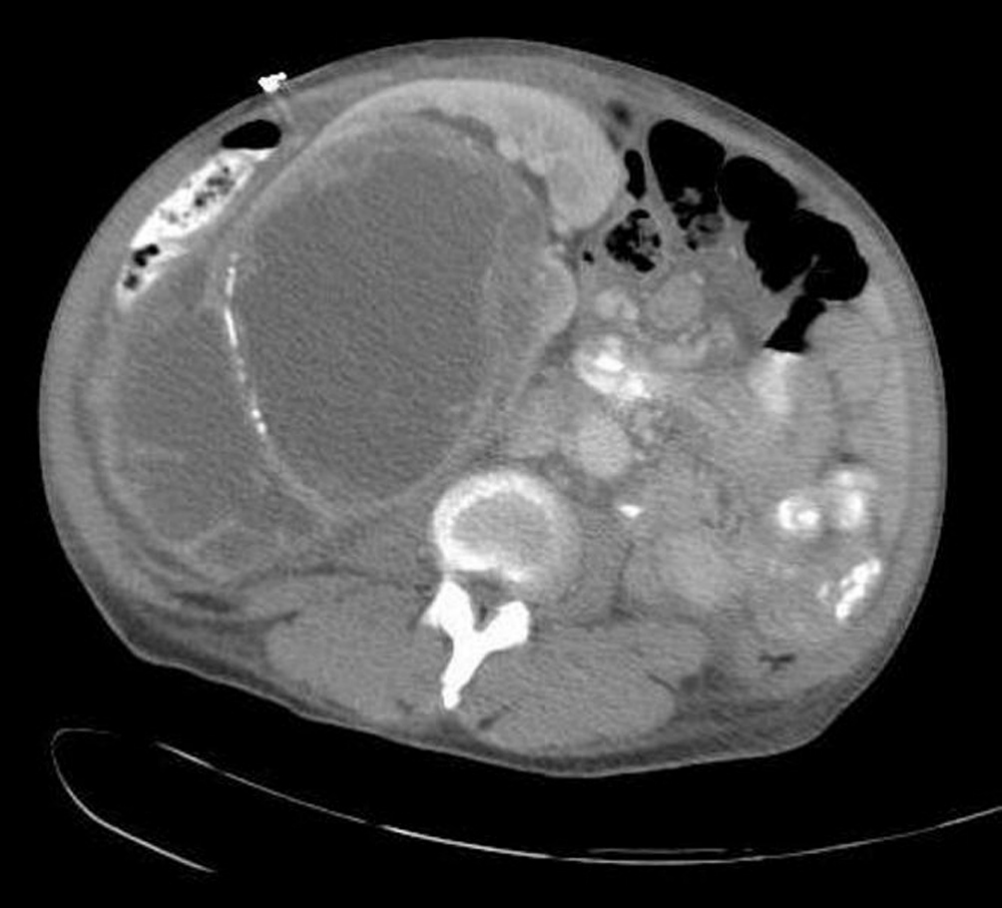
Abdominal CT scan revealing a large, complex cystic mass with multiple enhancing septations and septae calcifications arising from the right kidney.
